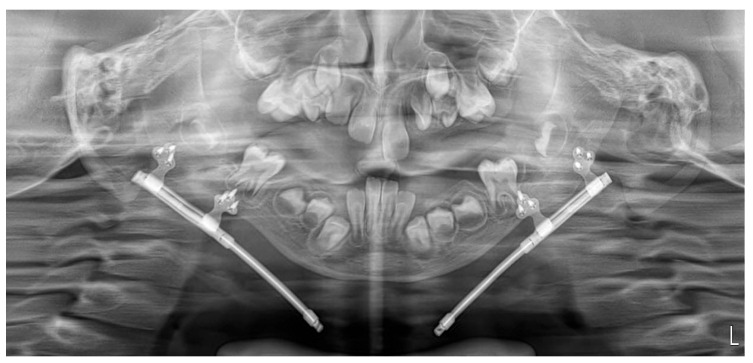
Panoramic radiograph obtained at 16 weeks after completion of distraction, immediately before removal of the distractors.
